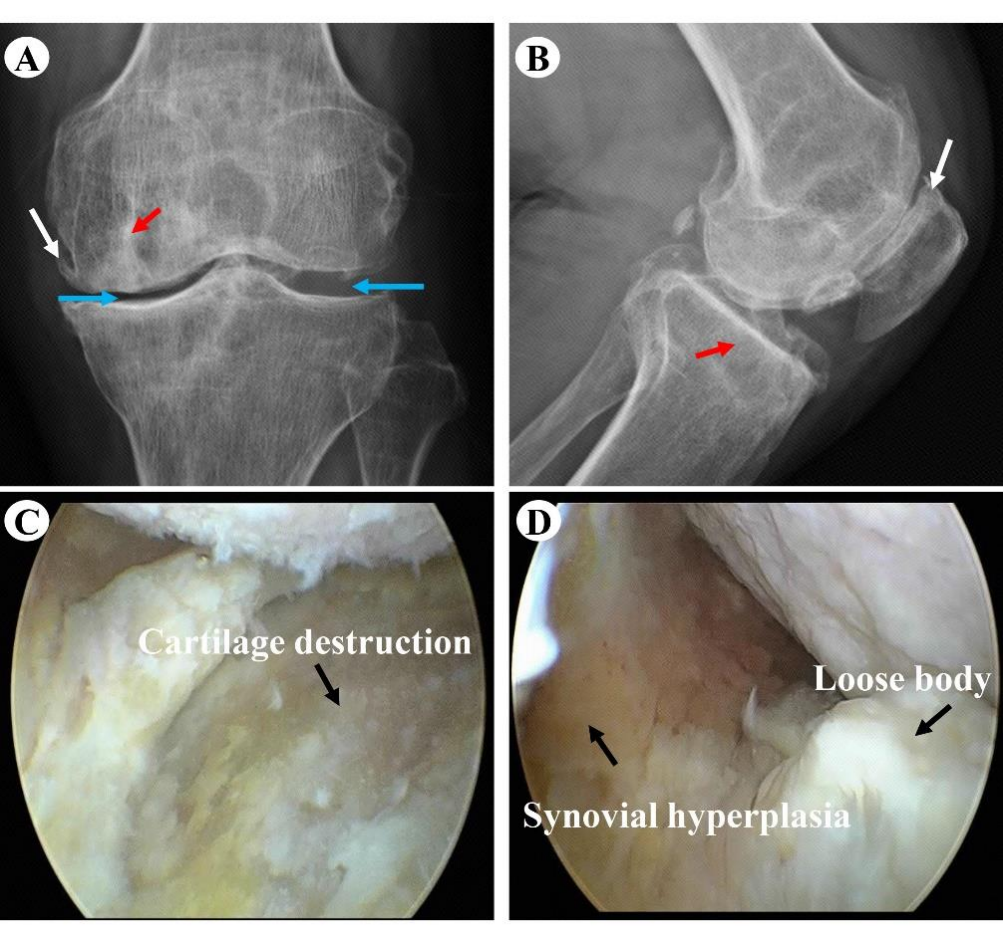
Radiographs and arthroscopy of osteoarthritis. *A* and *B* are anteroposterior and lateral radiographs, respectively. *C* and *D* are arthroscopy observations. A 57-year-old male patient came to see the doctor because a "sprain during exercise caused pain and discomfort in the left knee joint for one month." X-rays of the left knee showed narrowing of the medial space of the knee joint, hyperplasia of osteophytes, sclerosis of subchondral bone, and loose bodies in the intercondylar fossa. A CT scan was performed to further clarify the abovementioned lesions. Later, the patient underwent arthroscopic knee surgery. Intraoperative detection revealed that the cartilaginous surface of the left medial condyle of the femur and the medial plateau of the left tibia were severely damaged with arthritic synovial hyperplasia and a loose body in the intercondylar fossa. Red arrow: sclerosis of the subchondral bone in the left knee joint. Blue arrow: the internal joint space of the left knee is narrowed. White arrow: hyperplasia of osteophytes in the left knee joint.
